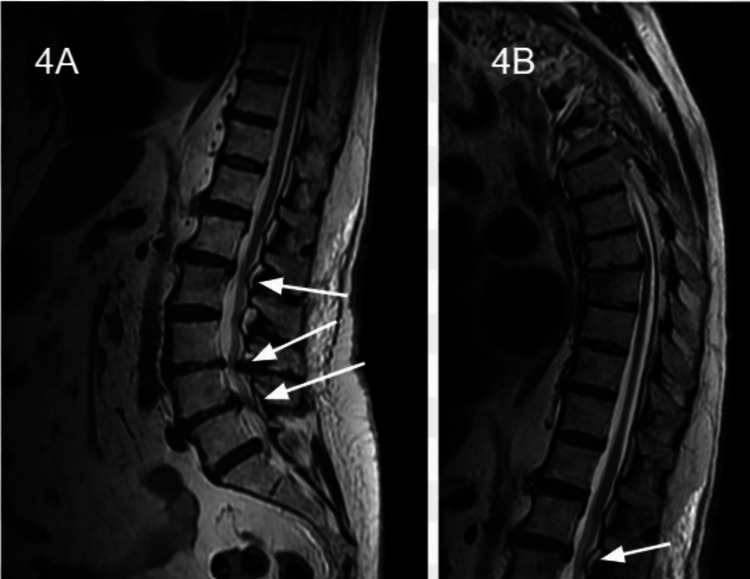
Sagittal MRI view of the thoracic and lumbar spine demonstrating spondylotic disease and disc disease causing mild cord compression at T5-T6 and T7-T8 without cord edema. No overall spinal canal or neural foraminal stenosis.
